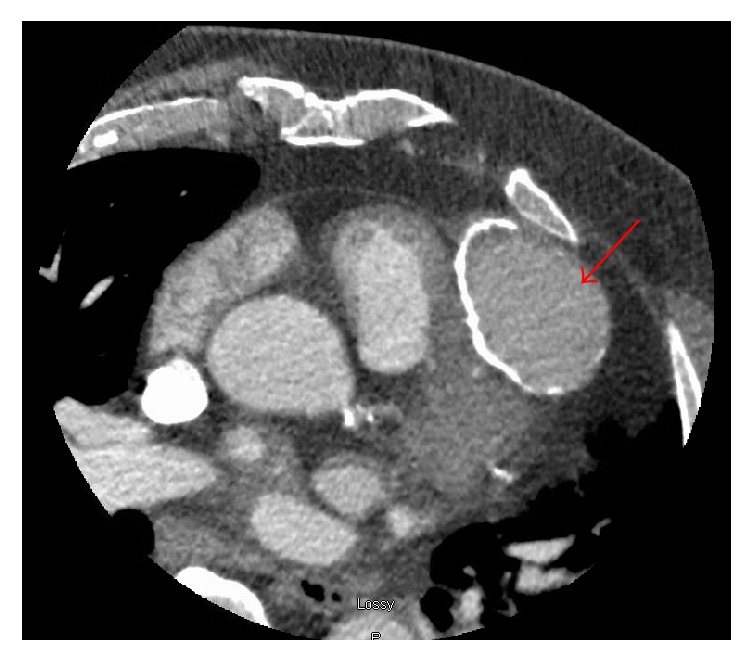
Cardiac CTA. Pseudoaneurysm arising superiorly from the mid-apical free wall of the right ventricle measuring up to 6.1 cm in maximal diameter with a 15 mm neck (red arrow).
